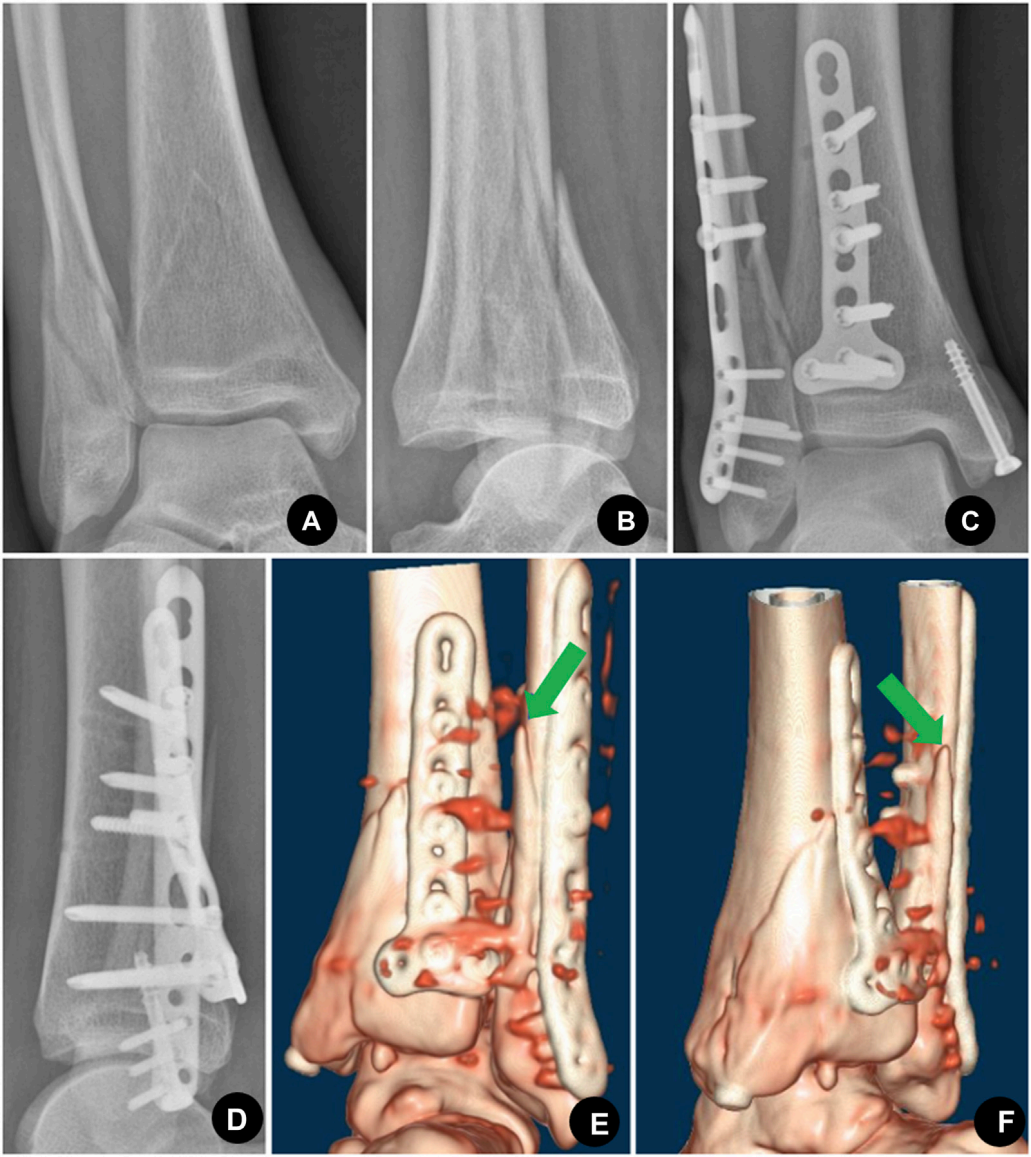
Sixty-seven-year-old female with trimalleolar fractures, the proximal apex of the lateral malleolus was located in zone III. (A), (B) Preoperative AP (antero-posterior) and lateral X-ray showed trimalleolar fractures with posterior dislocation of the ankle. (C), (D) Postoperative AP and lateral X-ray showed that the lateral malleolar fracture was fixed with the posterolateral antiglide-buttress plate, the medial malleolar was fixed with a cannulated compression screw, and the posterior malleolar was fixed with a T-shaped plate. (E), (F) Posterior and posteromedial view of the postoperative CT volume rendering showed that the distal fibular fracture apex was not compressed by the posterolateral plate as the apex (indicated by a green arrow in E and F) was located on the posterior ridge (zone III) which could not be reached by the pre-existing plate. As a result, the stability of the construct was impaired.
